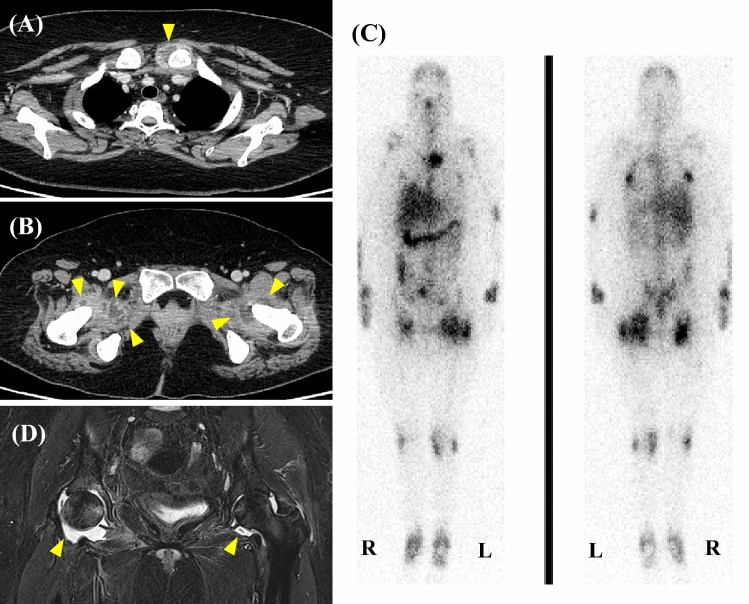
Radiological findings A, B Contrast-enhanced computed tomography showing effusions in the left sternoclavicular and both hip joints. C Gallium-67 scintigraphy demonstrating uptakes in bilateral wrist, knee, ankle joints, and large joints. D Magnetic resonance imaging of the hips detecting bone marrow edema along with fluid retention bilaterally
